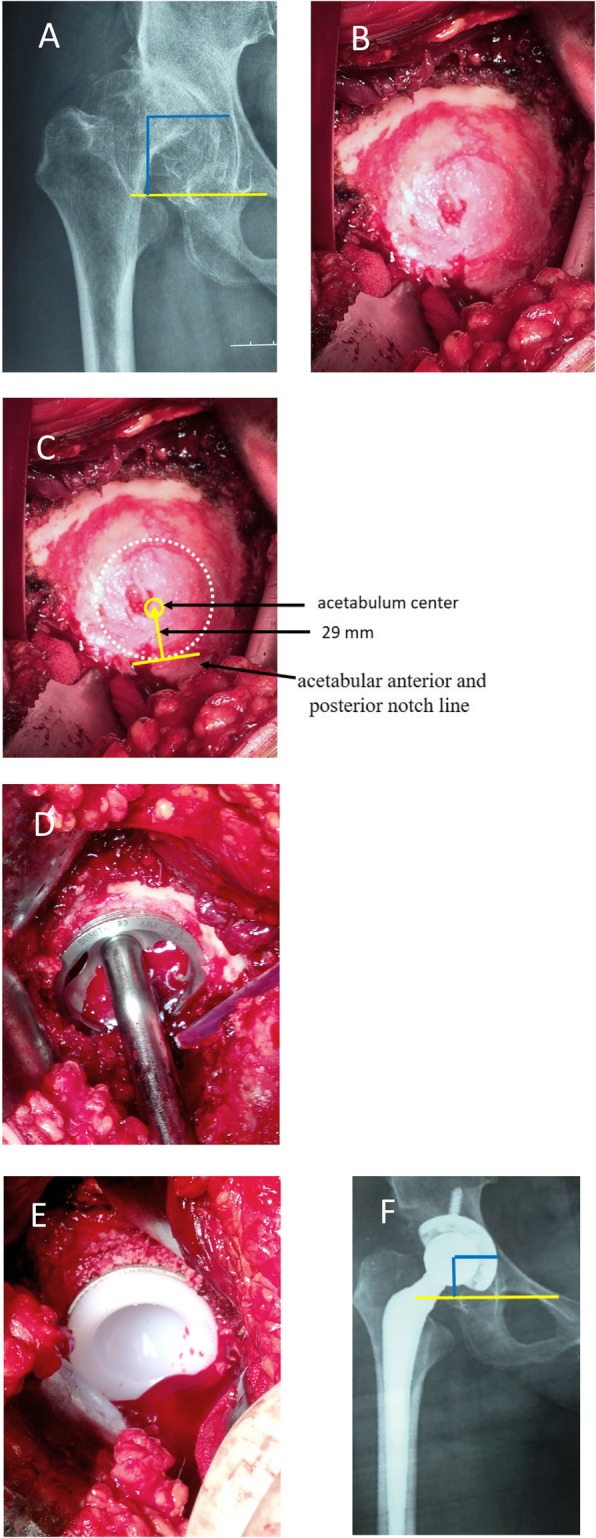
Radiographs of a 55-year-old male patient with end-stage osteoarthritis secondary to Crowe type II DDH. a Preoperative X-ray. b Intraoperative image showed dish-shaped acetabulum and closed shape Harris fossa. c Locating the acetabulum center. d Reaming the acetabulum and installing the test cup. e Installing the acetabular prosthesis. f Postoperative X-ray and rotation center restoration
